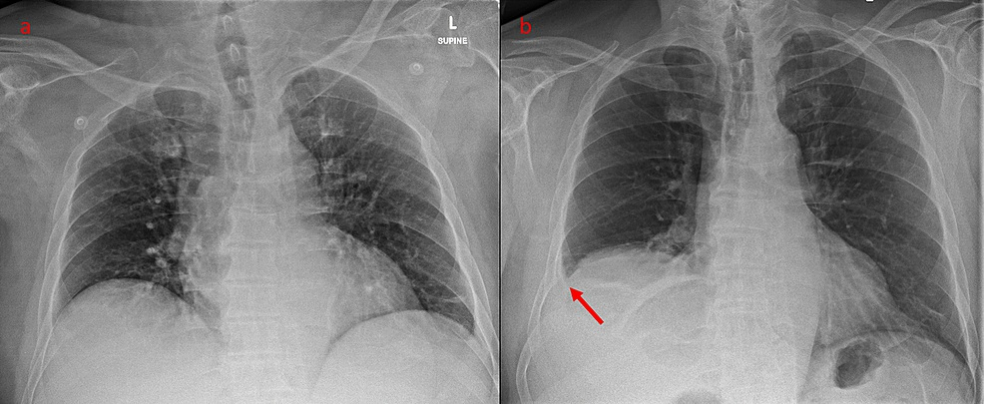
Supine anteroposterior CXR of Case 1 taken three years before admission (a), compared with erect posteroanterior CXR taken on admission (b), showing the development of elevated right hemidiaphragm and small right pleural effusion with basal atelectasis (arrow). CXR - chest X-ray
